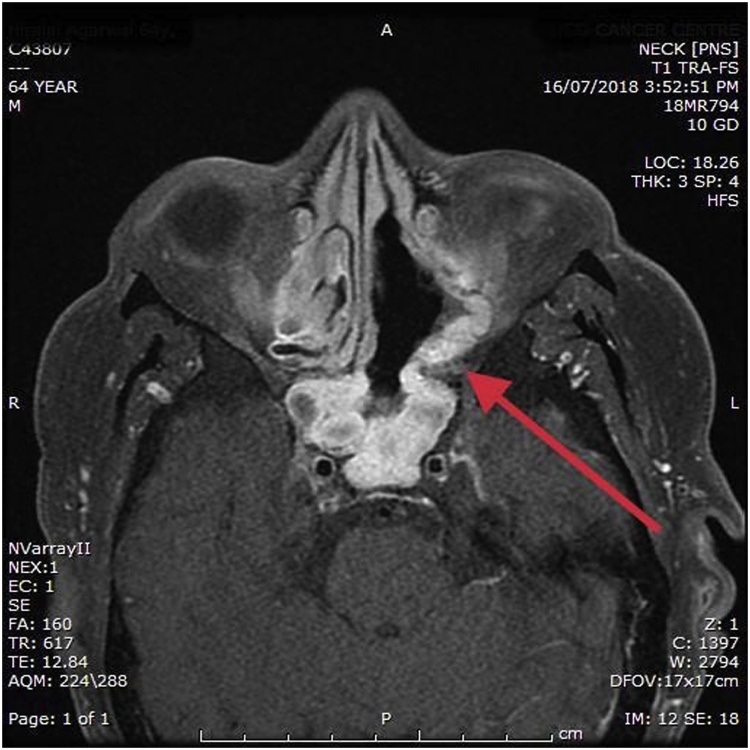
Post first surgery magnetic resonance imaging with contrast axial section of paranasal sinus.
